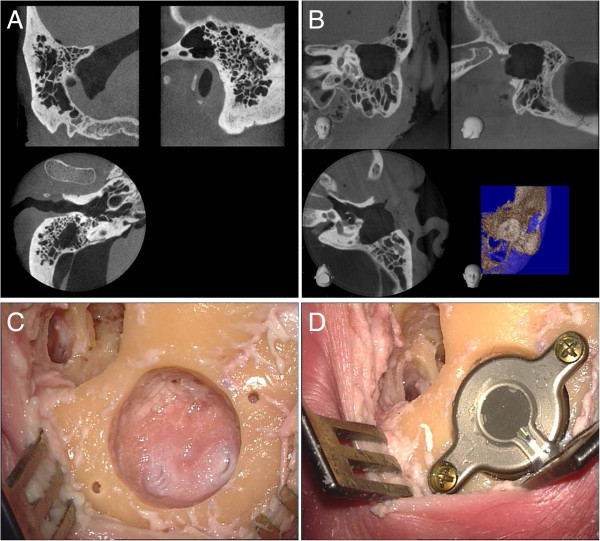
Preoperative CBCT (A and B) and intraoperative images (C and D). Preoperative radiological visualisation by CBCT of the site in the case of a well-pneumatised and not pre-operated mastoid (A) as well as a site with prior surgery in terms of a transcanalicular partial mastoidectomy (B). Presentation of the site after the drilling of the bed (C) and fixation of the implant (D).
